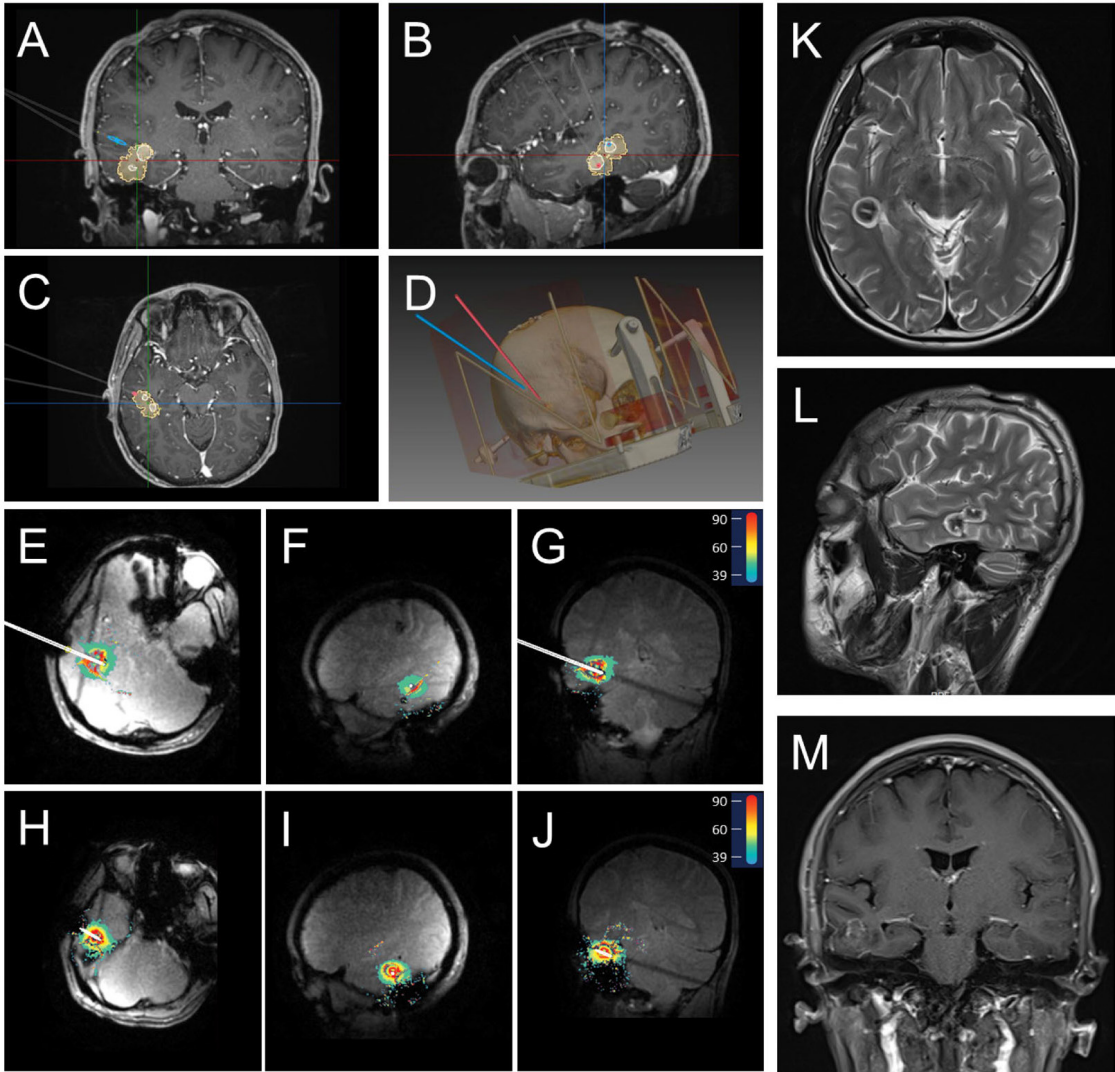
Representative CM patient's preoperative ablation trajectory planning, intraoperative ablation real‐time thermal monitoring, and postoperative MRI. This patient shows no typical EEG onset. (A–C) Ablation trajectory planning in coronal, sagittal, and axial plane. (White indicates the planned ablation and yellow indicates the actual ablation); (D) thin‐slice head CT with reconstruction; (E–G) ablation along the first trajectory in axial, sagittal, and coronal plane; (H–J) ablation along the second trajectory in axial, sagittal, and coronal plane; (K–M) postoperative 3‐month MRI in axial, sagittal, and coronal plane.
